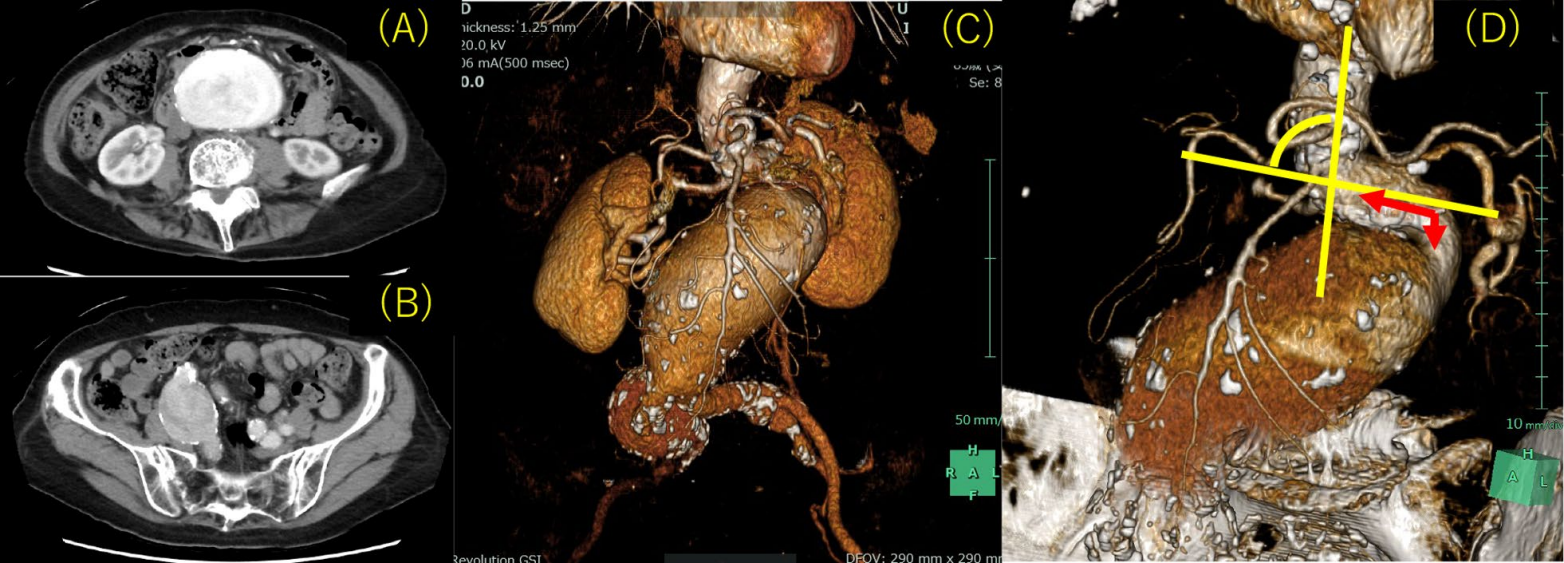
A Axial computed tomography images showing an abdominal aortic aneurysm. B Axial computed tomography images showing a right common iliac artery aneurysm. C Three-dimensional enhanced computed tomography image showing the presence of aneurysms in the abdominal aorta and right common iliac artery. D Preoperative volume rendering arteriography revealed that the neck angulation was 89.1 degrees, and the neck length was 17.9 mm
